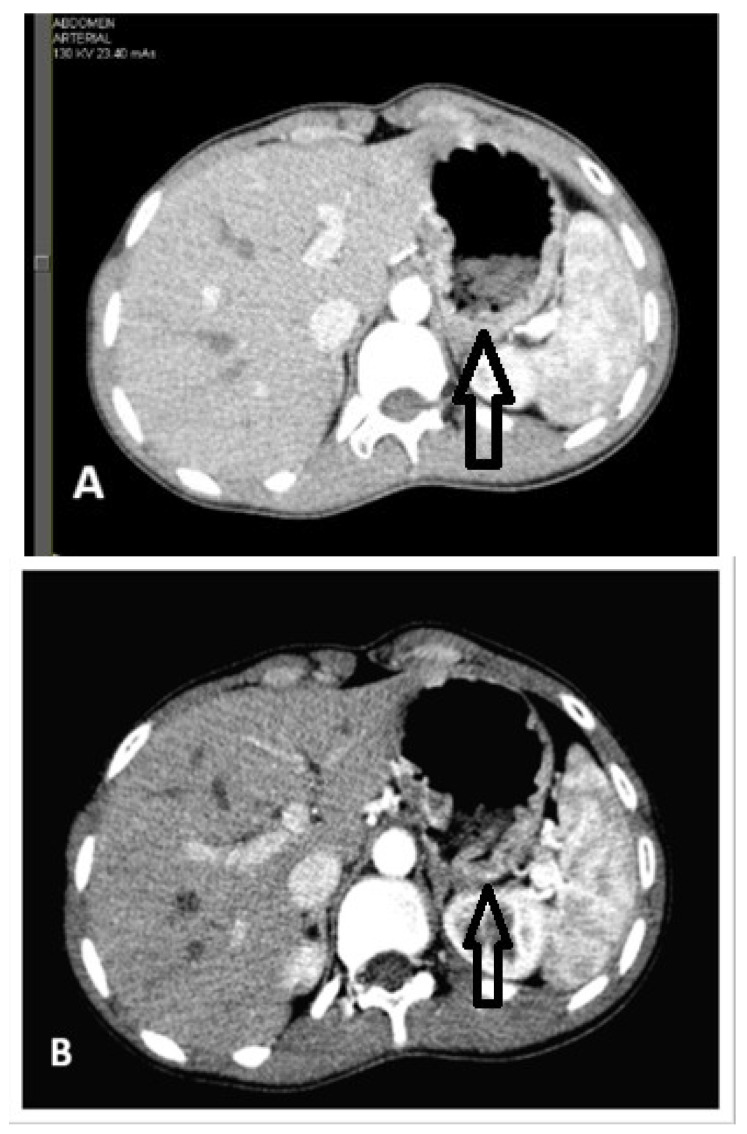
(A) Abdominal CT examination, arterial phase (arrow depicting moderate contrast intake and gastric wall thickening). (B) Abdominal CT examination, venous portal phase (arrow depicting gastric wall thickening).
